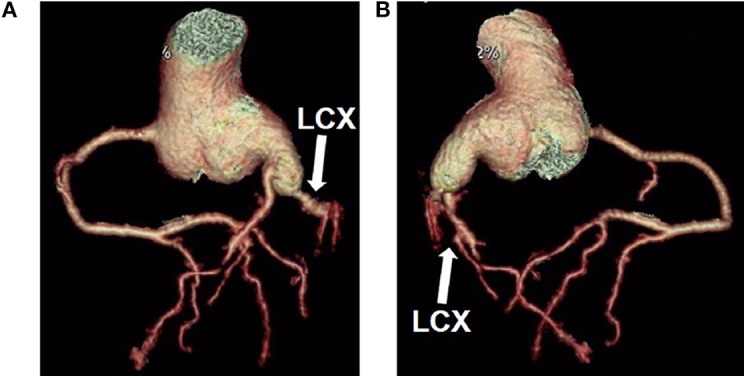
Cardiac computed tomographic showed constriction of the left coronary artery and circumflex artery (A,B). LCX, left circumflex artery.
